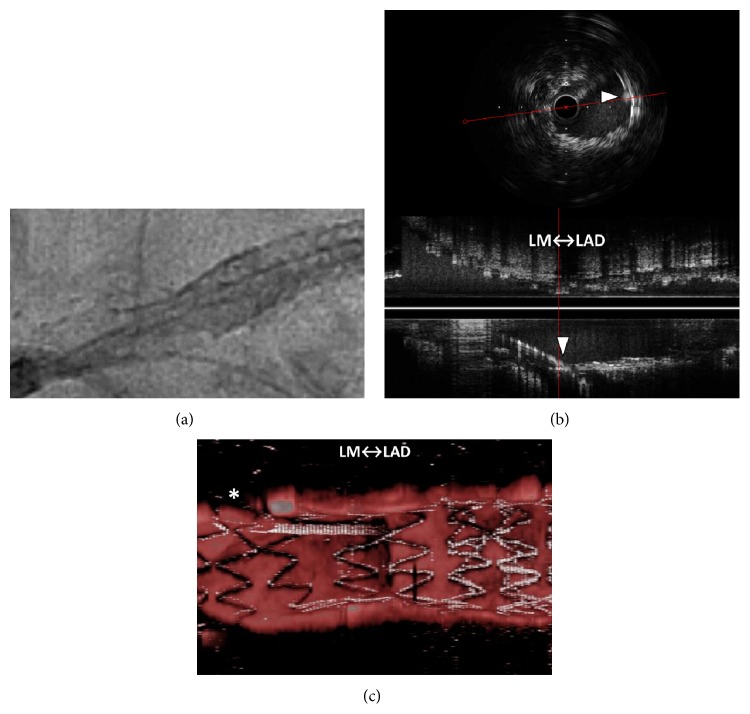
First rewiring. (a) X-ray fluorography, (b) intravascular ultrasonography showing the distal rewiring (arrowheads), and (c) intraprocedural instant stent-accentuated three-dimensional optical coherence tomography showing the rewiring through the smaller distal cell. LM: left main coronary artery, LAD: left anterior descending artery, and *∗*: guide wire shadow artifact.
